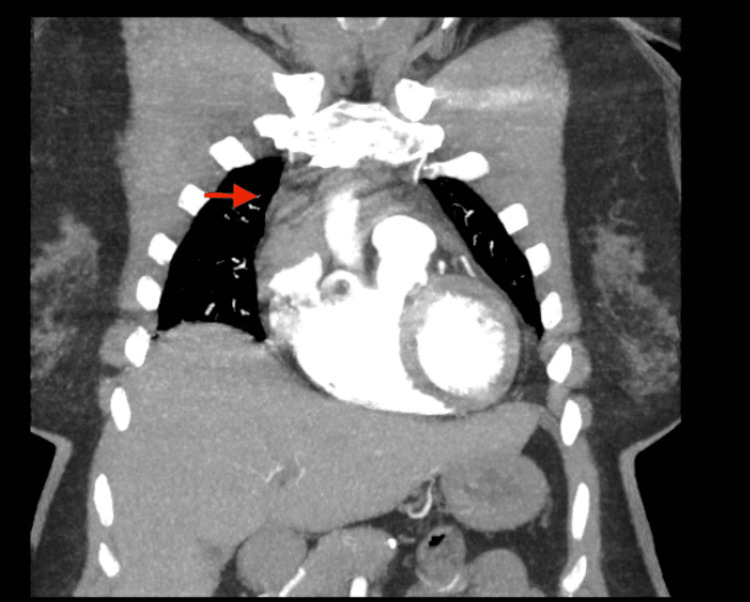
Coronal view CT chest. Red arrow indicating the anterior mediastinal hematoma.
